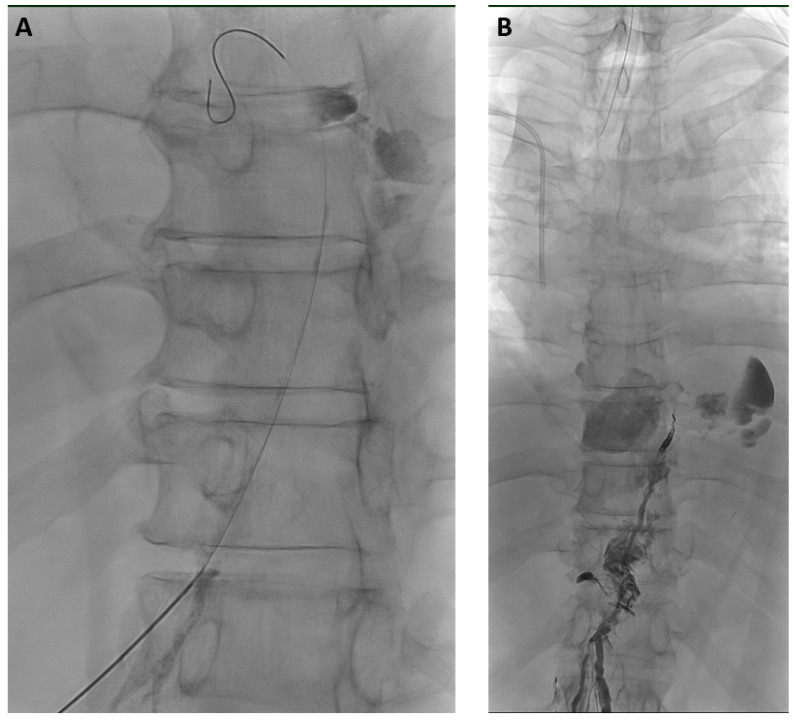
71-year-old man with bilateral chylothorax after esophagectomy. (A) X-ray lymphangiography demonstrated transection of the thoracic duct in the lower thorax with active leakage of contrast agent. After puncture of the thoracic duct, the inserted micro-wire already exits the thoracic duct at the leakage-site. (B) After thoracic duct embolization with micro-coils and a mixture of NBCA/iodized oil (ratio 1:2) leakage ceased immediately without recurrence or clinical sequelae over a follow-up time of 2.5 years.
